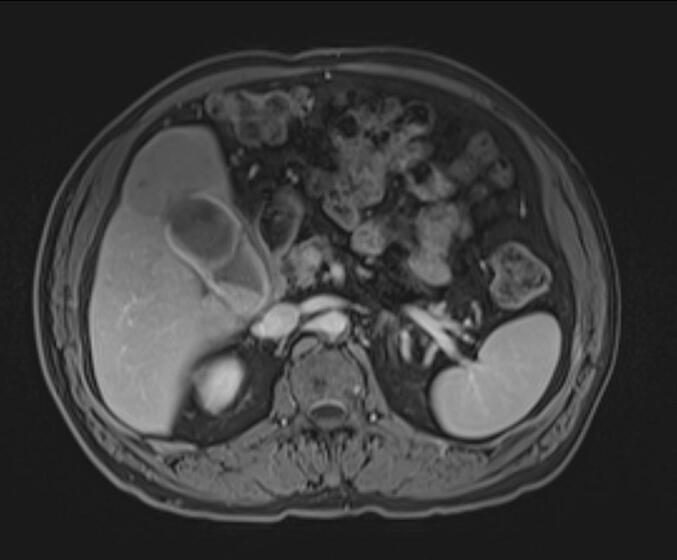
Axial T1-weighted contrast-enhanced MRI showing a heterogeneous gallbladder mass with liver invasion (segments IV and V), radiologically mimicking gallbladder cholangiocarcinoma.
